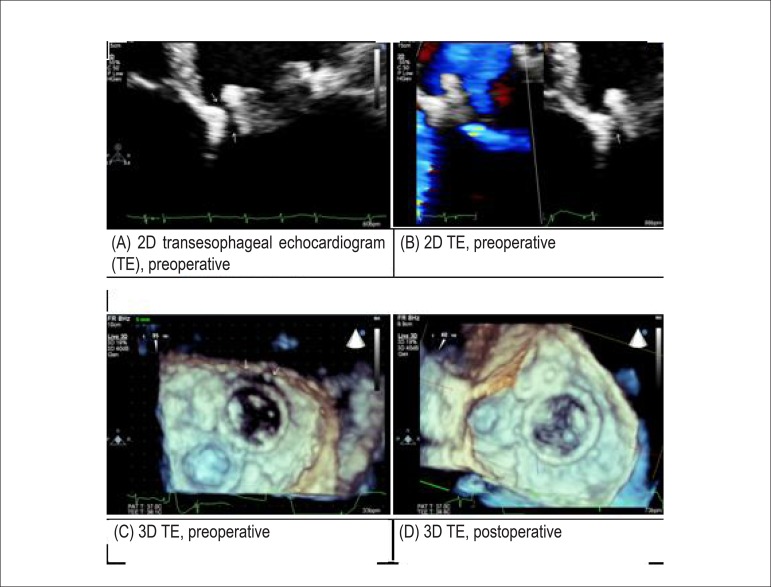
(A) Two-dimensional transesophageal echocardiogram (2D-TEE). (A) Preoperative
